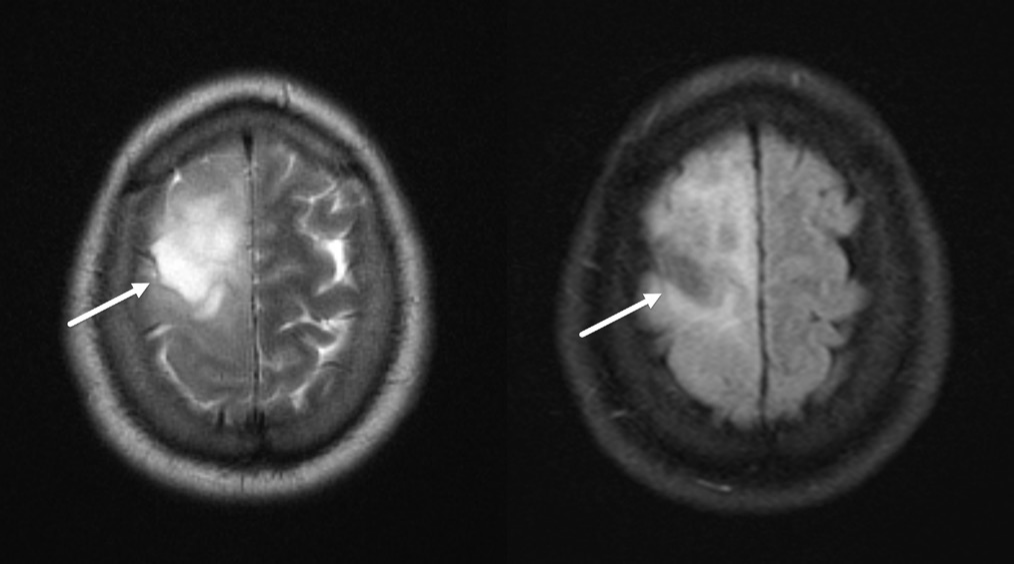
T2-FLAIR mismatch sign in IDH-mutant 1p/19q non-codeleted glioma. Axial T2 (left) and FLAIR (right) MR images demonstrate a large T2 hyperintense lesion in the right frontal lobe, which demonstrates FLAIR signal suppression (arrows), typical of the T2-FLAIR mismatch sign. FLAIR, fluid attenuated inversion recovery; IDH, isocitrate dehydrogenase.
